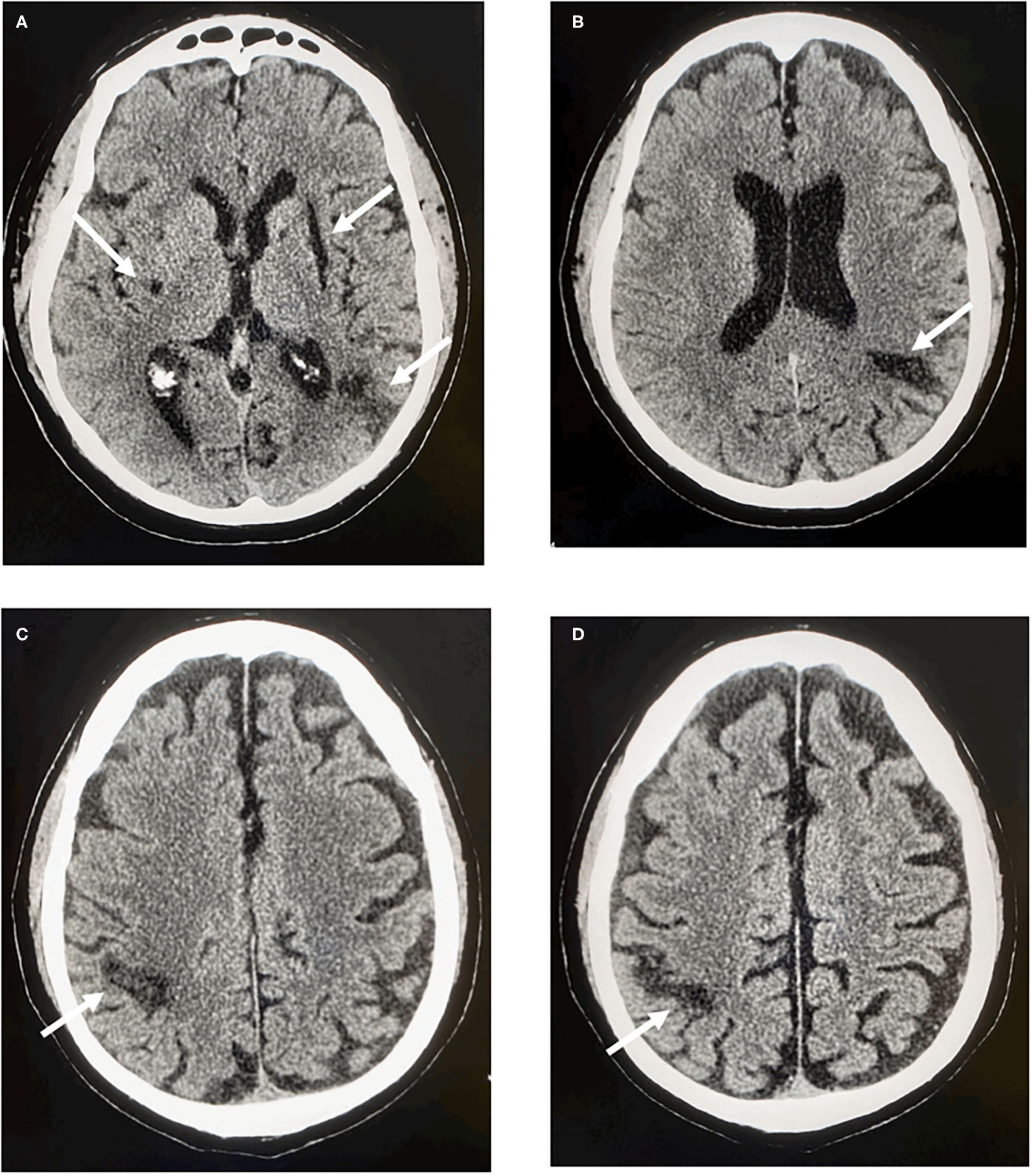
CT scan of the brain. (A) Left side: cortico-subcortical temporo-parieto-insular hypodensity. Right side: subcortical lenticular hypodensity. (B) Left side: cortico-subcortical parietal hypodensity. (C,D) Right side: cortico-subcortical parietal hypodensity.
